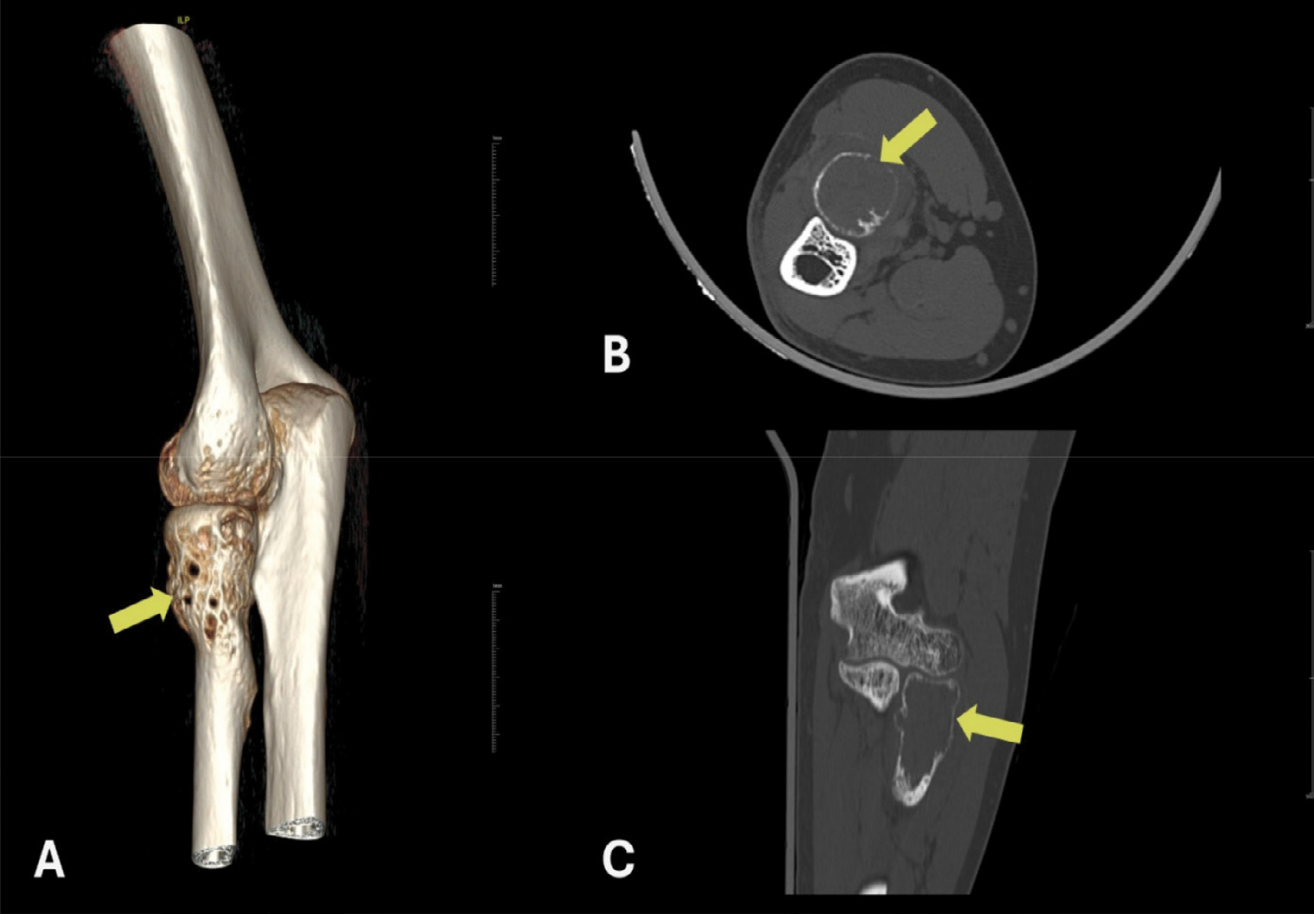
Three‑dimensional reformatting (A), axial (B), and coronal (C) CT images demonstrate a well‑defined, lytic and expansile bone lesion located at the proximal metadiaphyseal region of the radius. The lesion is associated with cortical thinning (indicated by yellow arrows).
